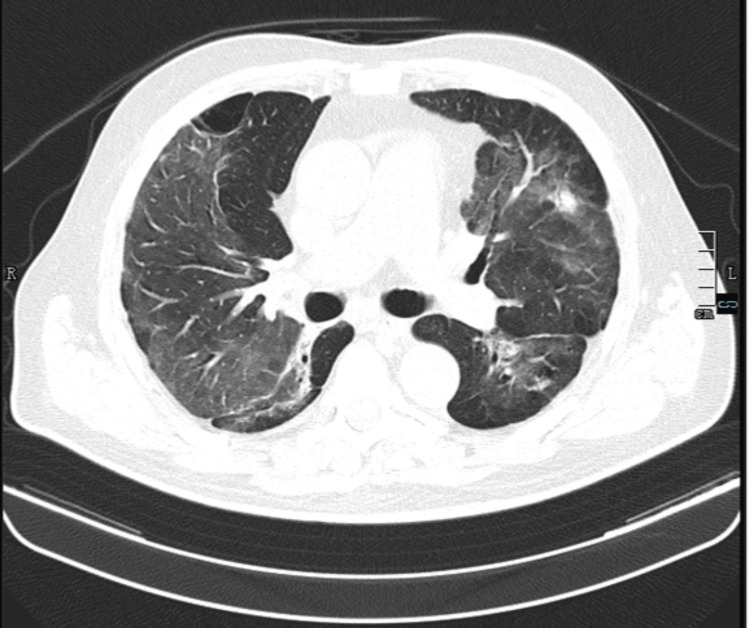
Chest CT (after 14 days of corticosteroid therapy) Lung window, axial view. CT demonstrating significant regression of bilateral infiltrates compared to the previous examination. CT: computed tomography
